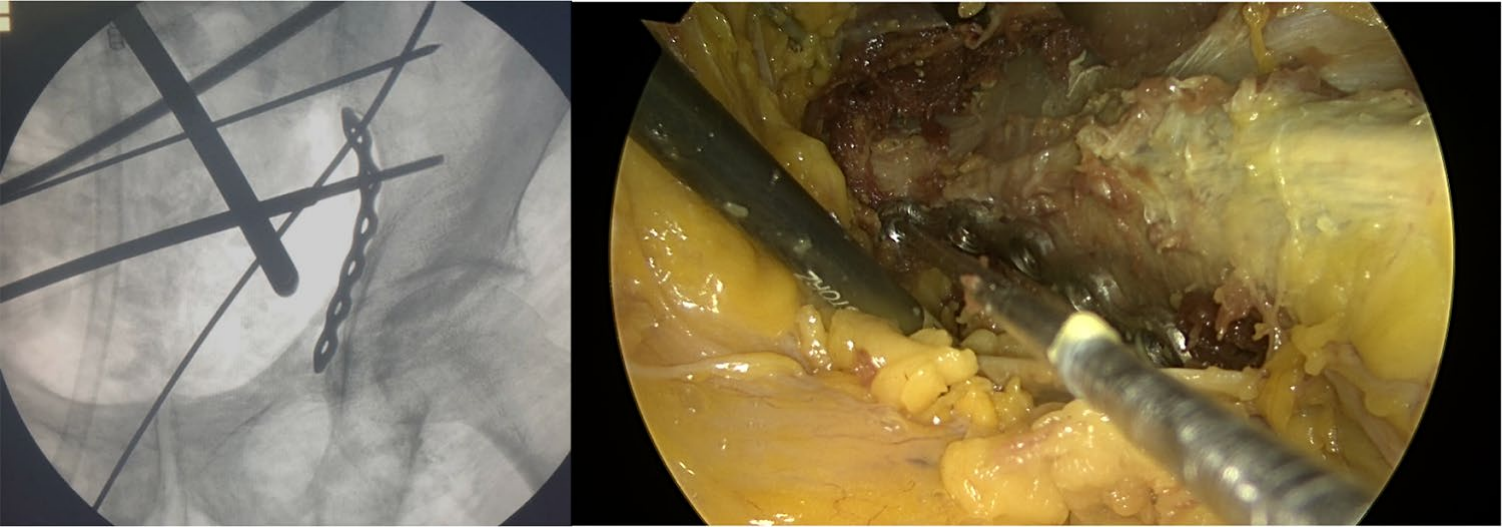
Shows an example of a plate placed to the aspect below the pelvic rim with a corresponding radiograph
